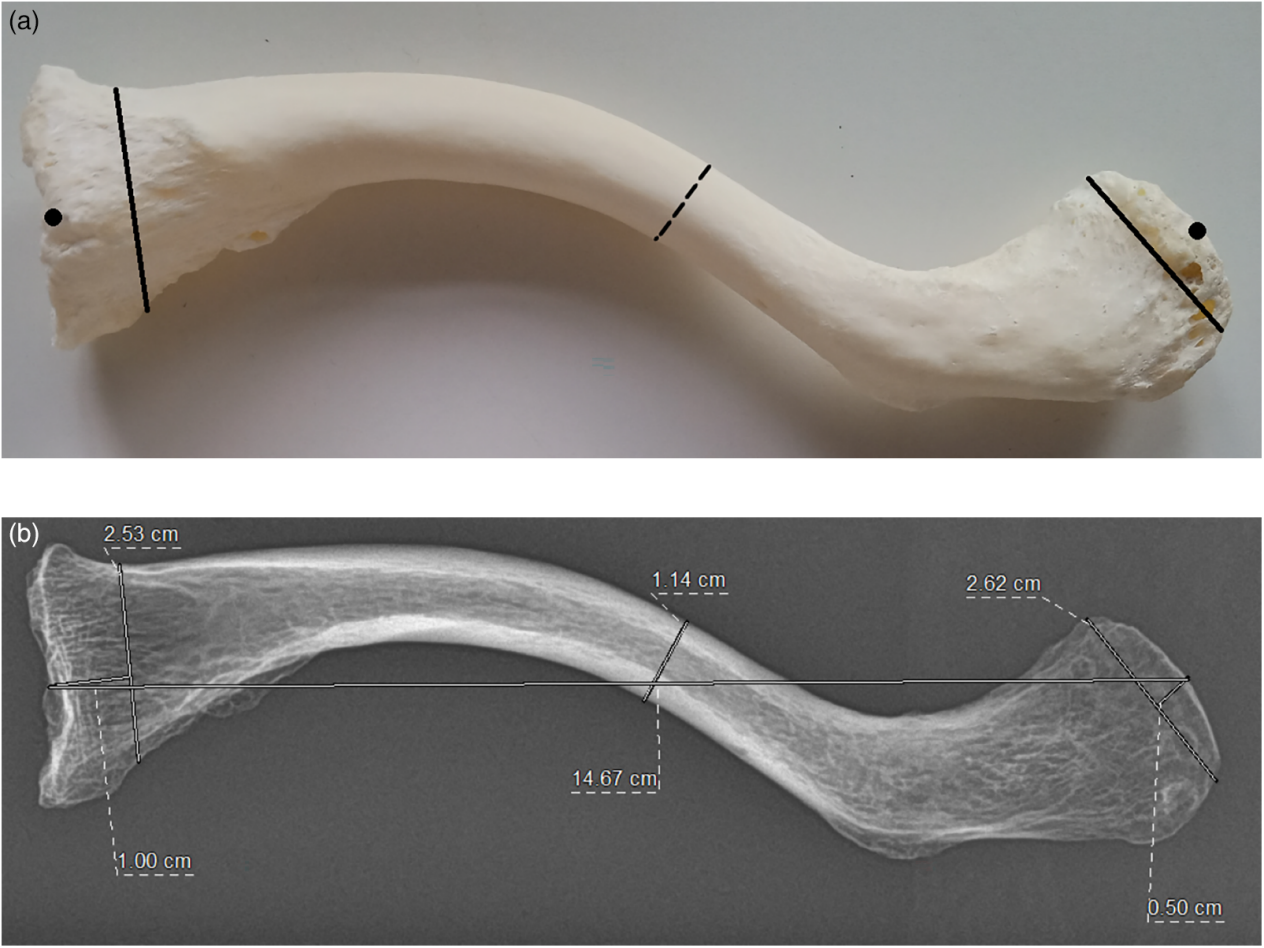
(a) The measurement protocol for manual assessment of “clavicle 1” was defined as follows: clavicular length was measured as the linear distance between the medial and lateral articular centers using precision calipers and a ruler (•). Medial clavicular width was assessed approximately 1 cm distal to the sternal end (−), while lateral clavicular width was measured 0.5 cm proximal to the acromial end (−). The central width was determined by identifying the midpoint between these two measurement points (…). (b) The measurement of the relevant anteroposterior x-ray images was performed using RIS/PACS. The macerated clavicles were assessed following the method described in (a).
